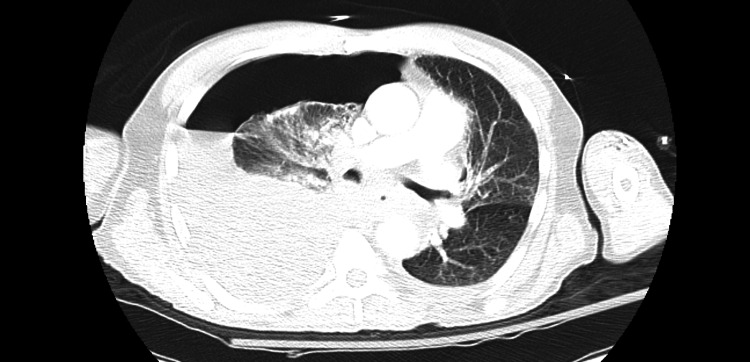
CT angiogram of the chest showing right-sided pleural effusion
